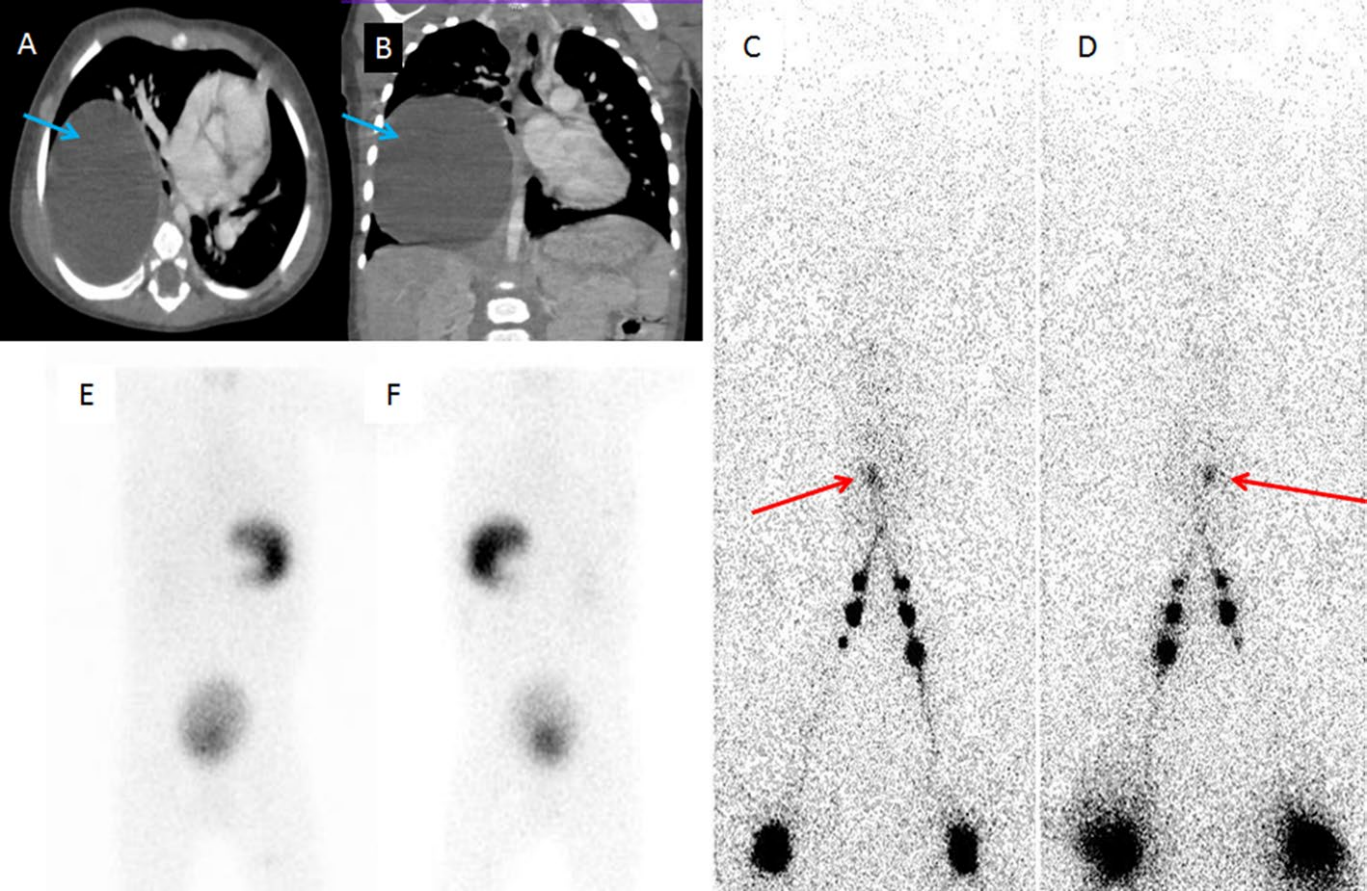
Transaxial and coronal preoperative CT image showing the oval to round shaped large cystic lesion (A,B). Anterior (C) and posterior (D) whole body images acquired 30 min post intradermal injection of filtered Tc99m sulphur colloid in the the first interdigital webs of bilateral lower limbs. Focal accumulation of radiotracer seen in the right lower hemithorax region (as shown by red arrow). No abnormal foci of radiotracer uptake seen in the neck and thorax region on anterior (E) and posterior (F) images acquired after ointravenous injection of 2 mCi of Tc99m pertechnetate to suggest heterotrophic gastric mucosa. Tc99m pertechnetate images were acquired after 48 h of Tc99m sulphur colloid lymphoscintigraphy
